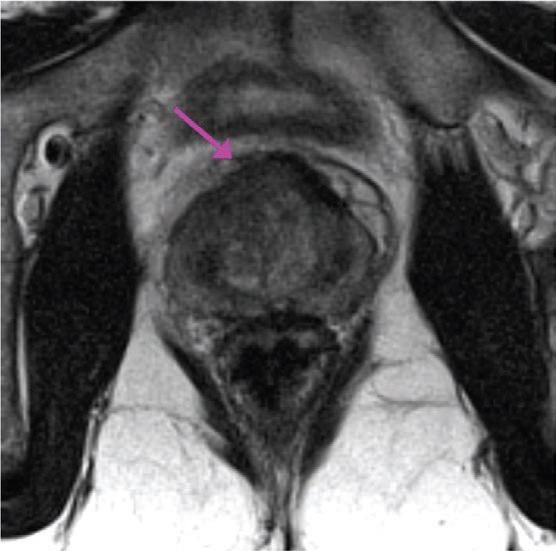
High-resolution T2-weighted image of the prostate. PCa appeared as a lenticular low-signal intensity lesion of the anterior fibromuscular stroma infiltrating into the transitional zone.
